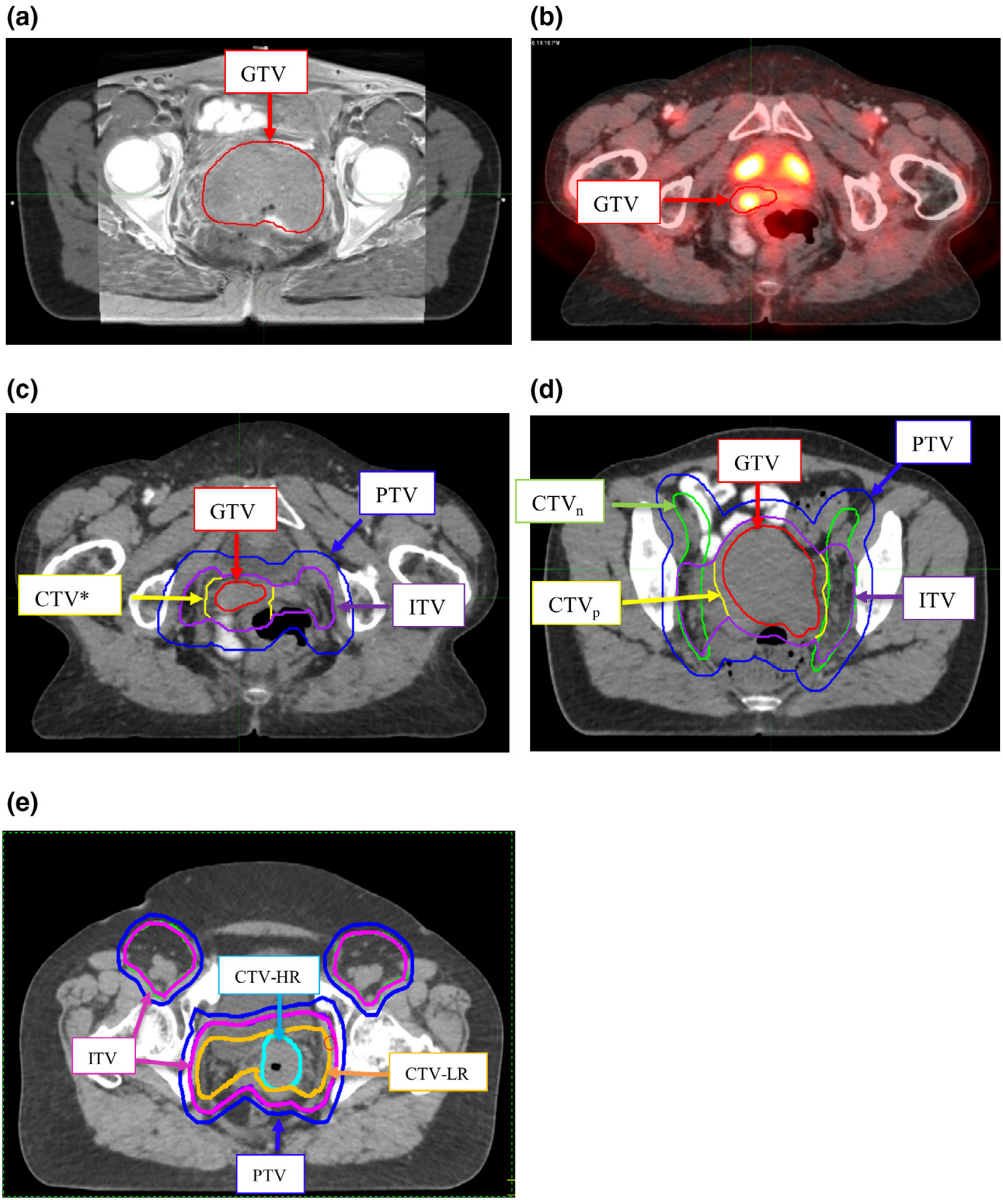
Target contouring. (A) MRI/CT delineation of GTV in cervical example. (B) PET/CT delineation of GTV in vaginal example. PET and/or MRI may be used in both cervical and vaginal cancer cases. (C) Target contours in vaginal case. CTV = GTV + 0.7 cm. ITV = GTV + 0.5 cm. PTV = CTV/ITV + 1 cm. (D) Target contours in standard cervix case. CTV = (seroma + 0.5 cm) + (GTV + 0.5 cm for VMAT / +1 cm for conformal). PTV = ITV/CTV + 1 cm. (E) Target contours in EMBRACE‐II cervix case. PTV = ITV + 0.5 cm. CT = computerised tomography, CTV = clinical target volume, CTV‐HR = high‐risk CTV, CTV‐LR = low‐risk CTV, CTVn = nodal CTV volume, CTVp = primary CTV volume, GTV = gross tumour volume, ITV = internal target volume, MRI = magnetic resonance imaging, PET = positron emission tomography, PTV = planning target volume, VMAT = volumetric modulated arc therapy.
